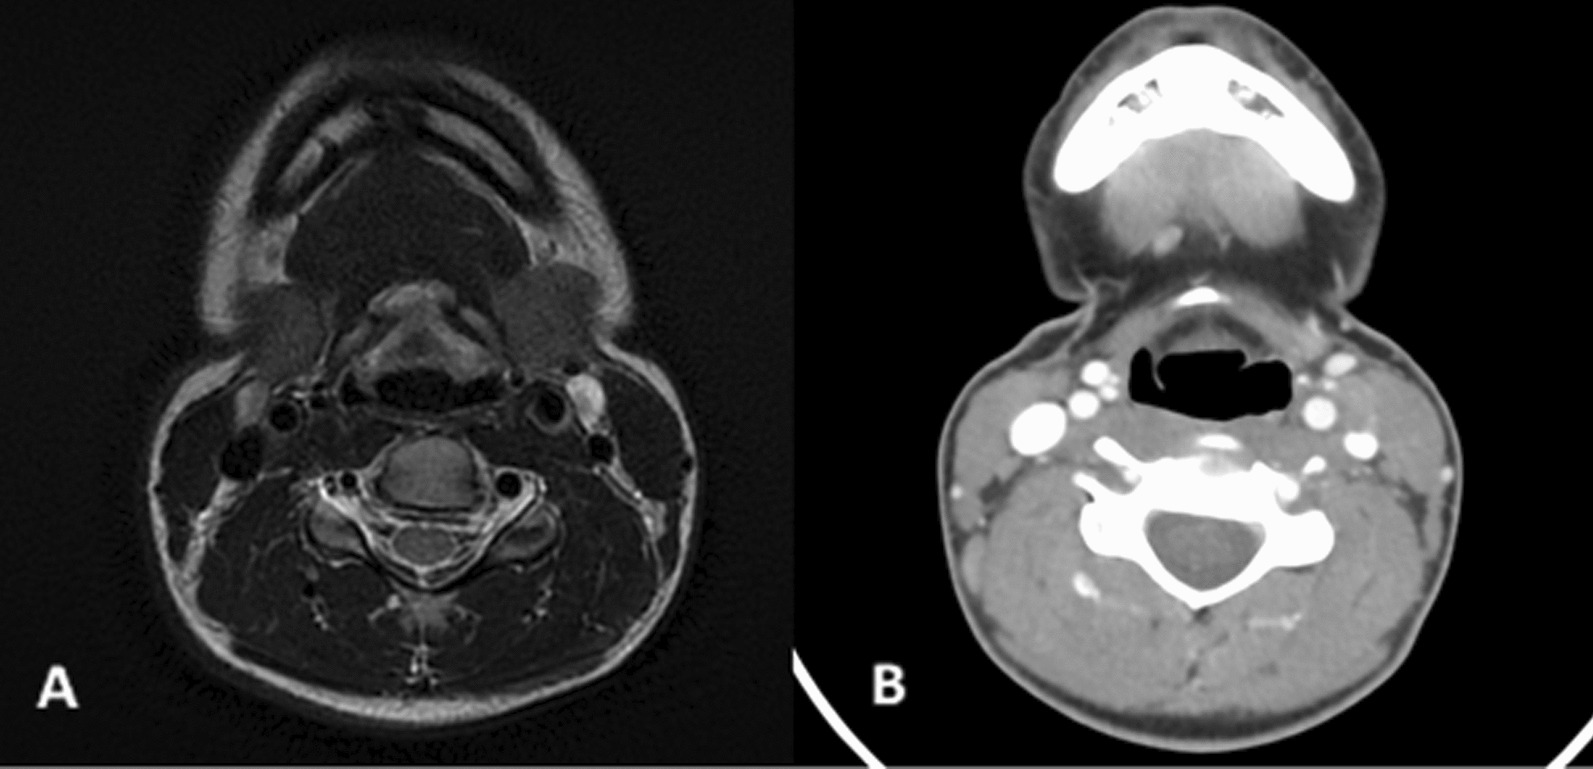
A Neck MRI (T2 fat saturation) and B injected neck CT scan confirmed the presence of supracentimetric bilateral neck node disease
